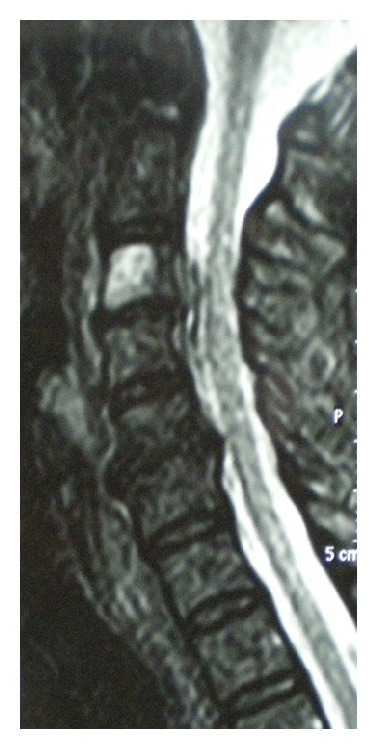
Preoperative MRI in sagittal plane demonstrating the spinal cord compression from a ventral C3-C4 epidural abscess.
